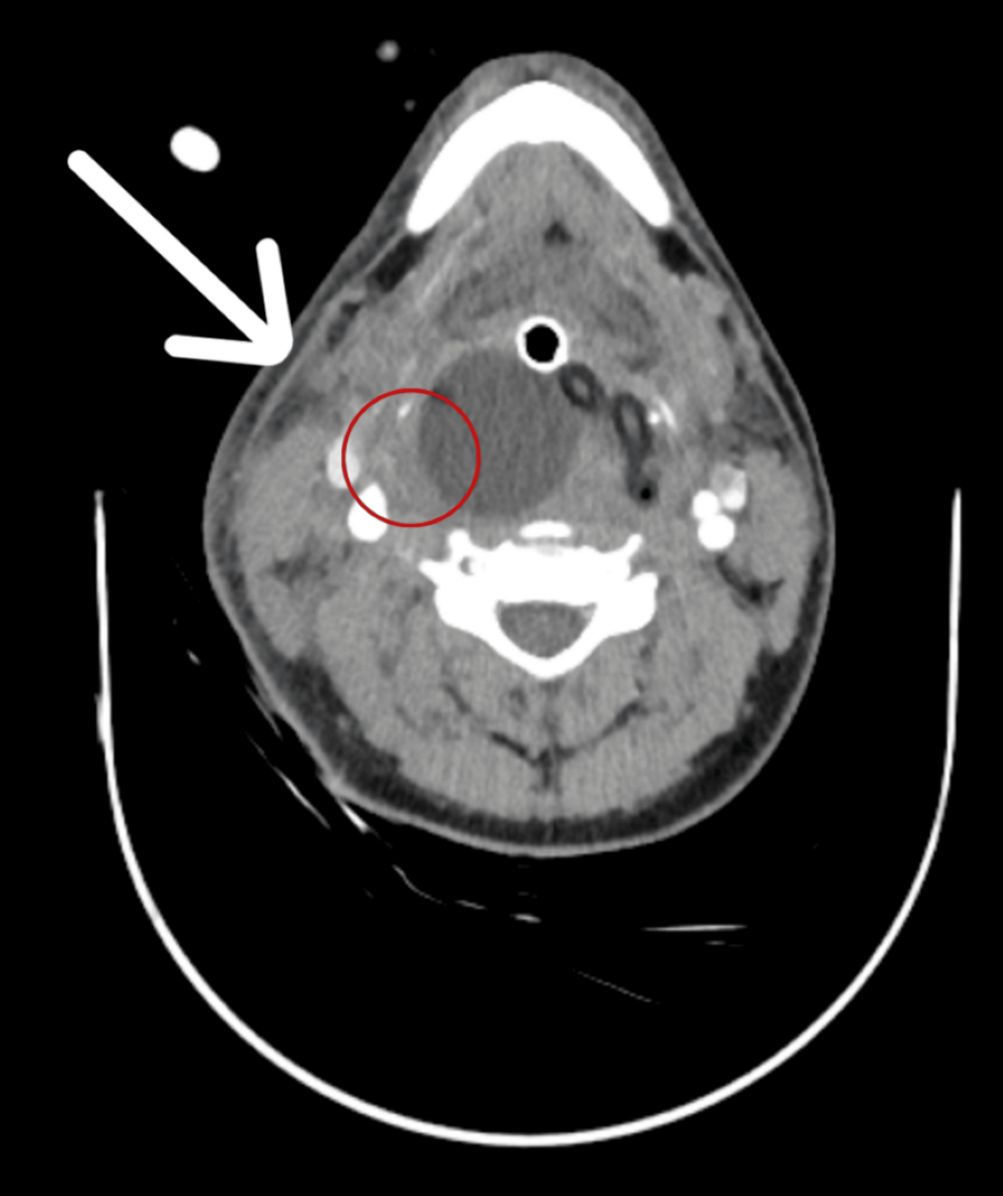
CTA neck after 72 hours. ‏CTA neck after 72 hours showing marked regression of the right-sided hematoma and complete resolution of contrast extravasation (arrow, red circle). CTA, computed tomography angiography
